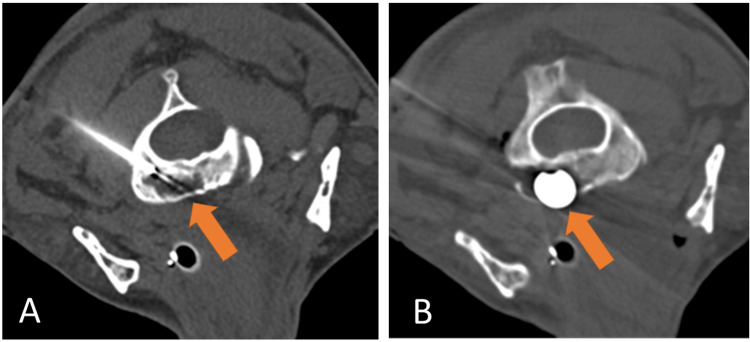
Intraoperative images during C2 lesion biopsy and kyphoplasty. A) axial bone window CT image showing posterior approach access of the C2 body with biopsy device deployed. B) axial bone window CT image showing balloon tamp reduction for cavity creation.
